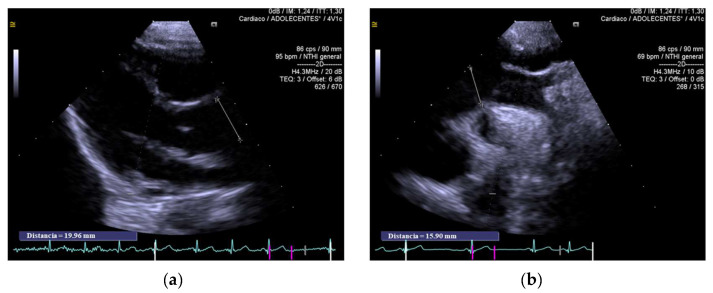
Ascending aortic dilatation in individual I4. (a) The parasternal long-axis view of the ascending aorta, showing a mild dilated aorta. Aortic ring, 16.5 mm (z + 3.39); sinus of Valsalva, 22.6 mm (z + 3.06); ascending aorta, 19 mm (z + 2.92). (b) Suprasternal view of aortic arch.
